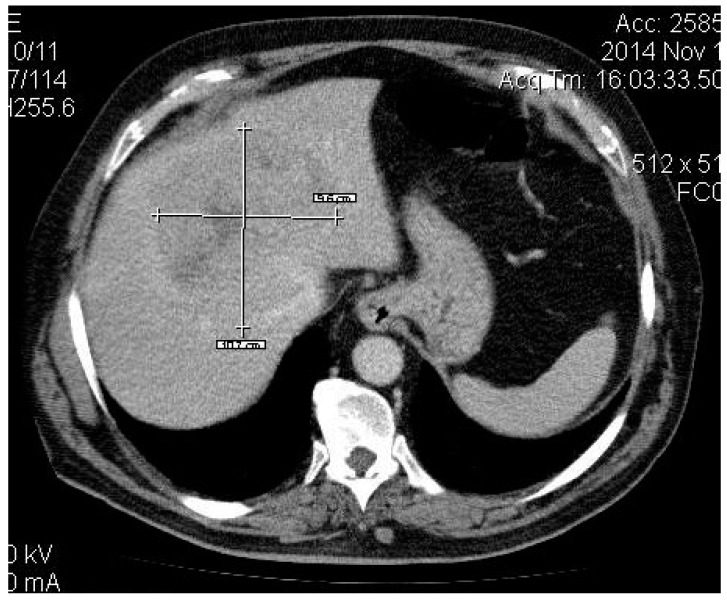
CT revealed a giant metastatic lesion located centrally, mainly in segment 4.
